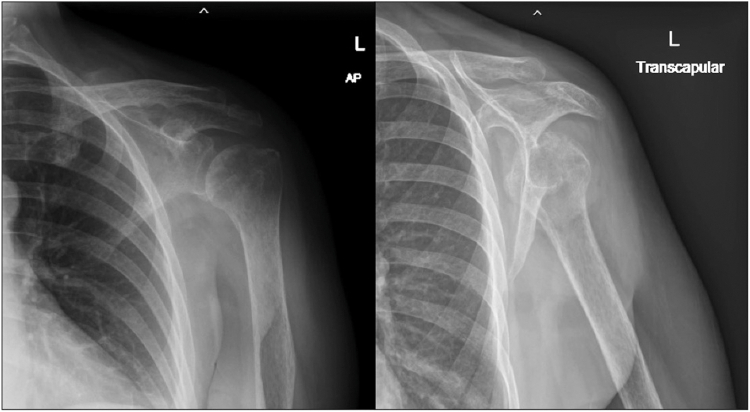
Follow-up plain radiographs showing maintained reduction and normal alignment of the glenohumeral joint, with no residual inferior subluxation. *AP*, anteroposterior.
